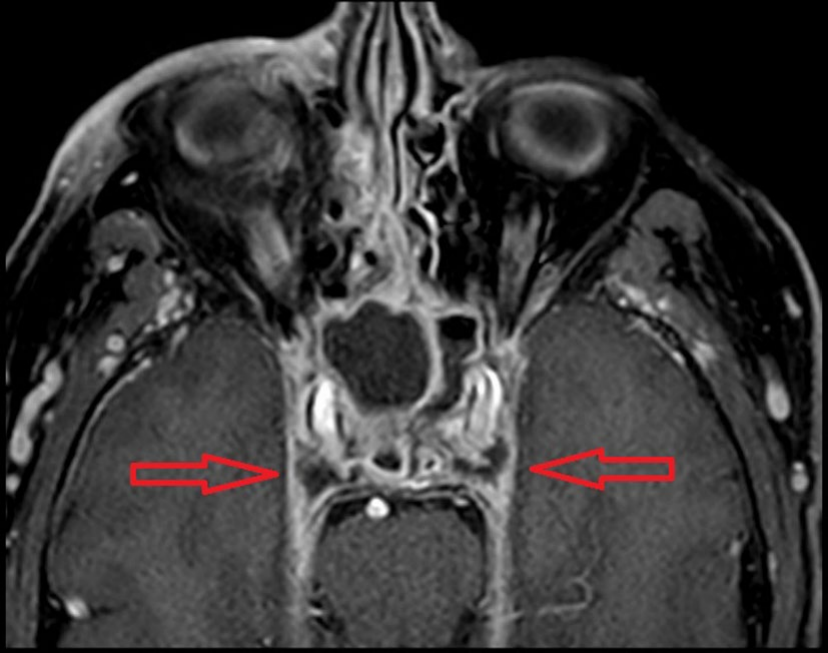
Contrast-enhanced MRI (3D T1GE; E-THRIVE); bilateral filling defect in the cavernous sinus (red arrows) and bilateral opacification of the sphenoid sinus
